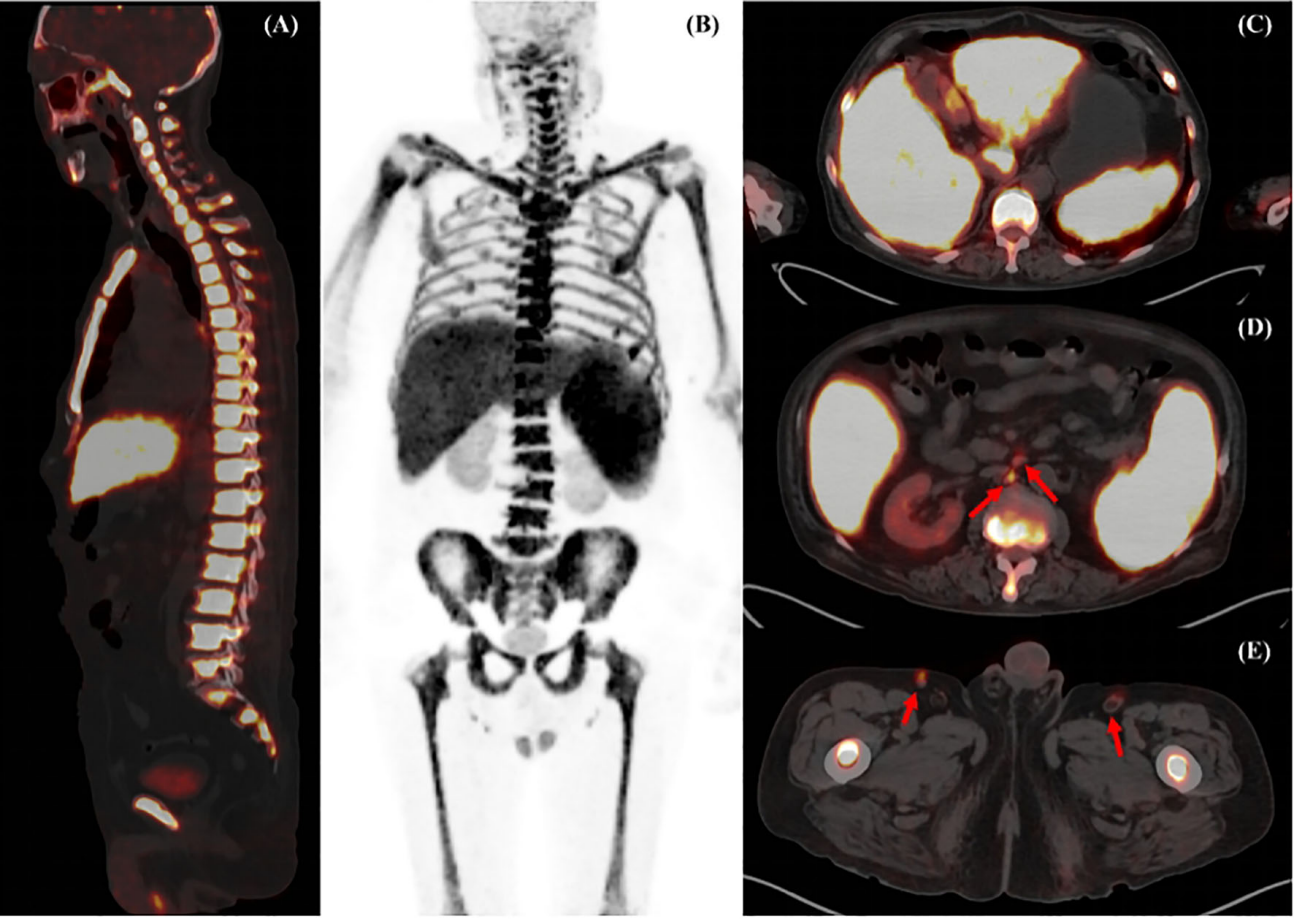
Maximum intensity projection (MIP) images of ^18^F-FDG PET/CT (A) showed markedly increased diffuse abnormal ^18^F-FDG uptake in the bone marrow of the axial and appendicular skeleton. Fusion PET/CT images (B) demonstrated the middle axis and sternum with high metabolic activity. Transverse fusion PET/CT showed increased uptake throughout the enlarged spleen and liver (C), and revealed increased FDG uptake in slightly enlarged lymph nodes in the retroperitoneal and bilateral inguinal areas (D, E, arrow).
